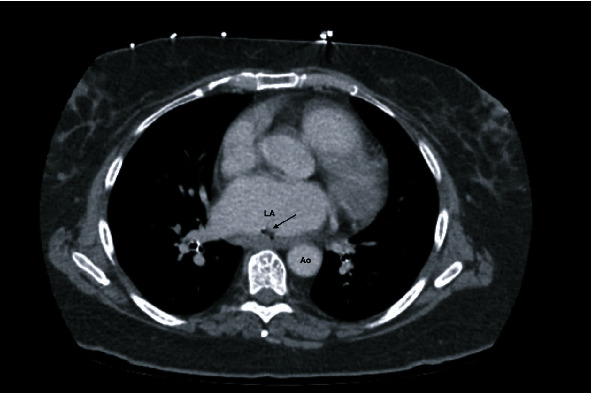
Cross-sectional image of CT scan with IV contrast demonstrating the atrio-esophageal defect. LA: left atrium; Ao: Aorta; arrow: extraluminal air adjacent to the esophagus.
